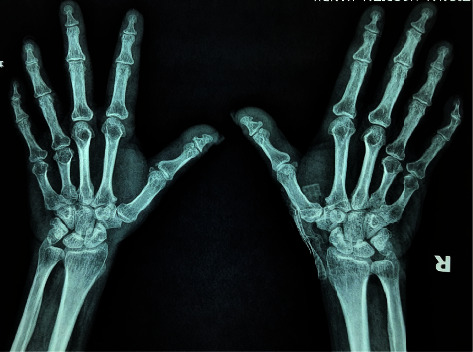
X-ray of bilateral hands showing brachymetacarpia.
